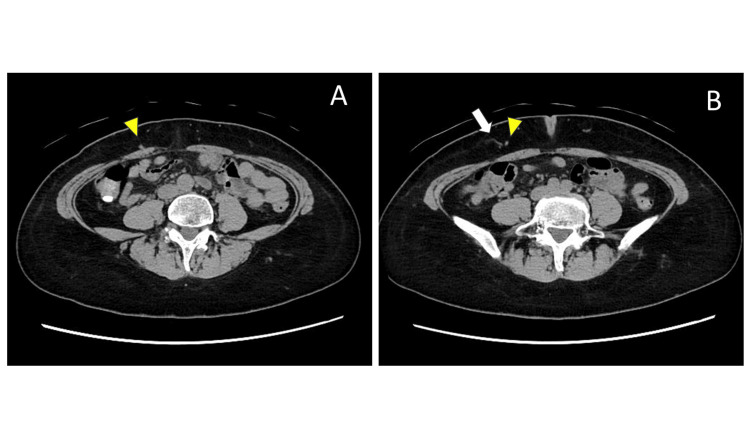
Computed tomography images of the abdomen. (A) An axial CT image obtained at the level of the patient’s maximal tenderness demonstrates a subtle linear structure extending from the rectus abdominis muscle toward the subcutaneous tissue (yellow arrowhead), consistent with the expected course of an anterior cutaneous nerve.
